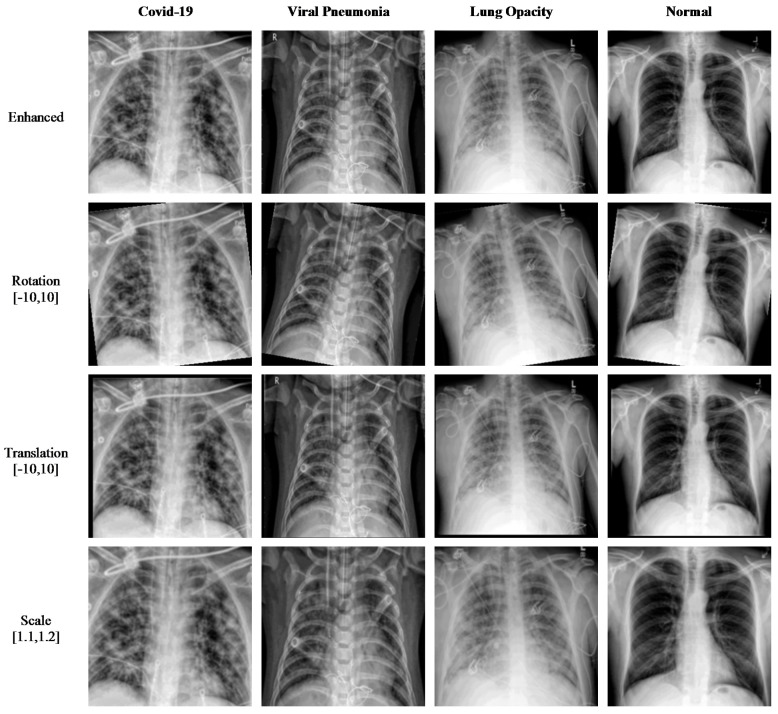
Sample of X-ray images produced using data augmentation methods.
